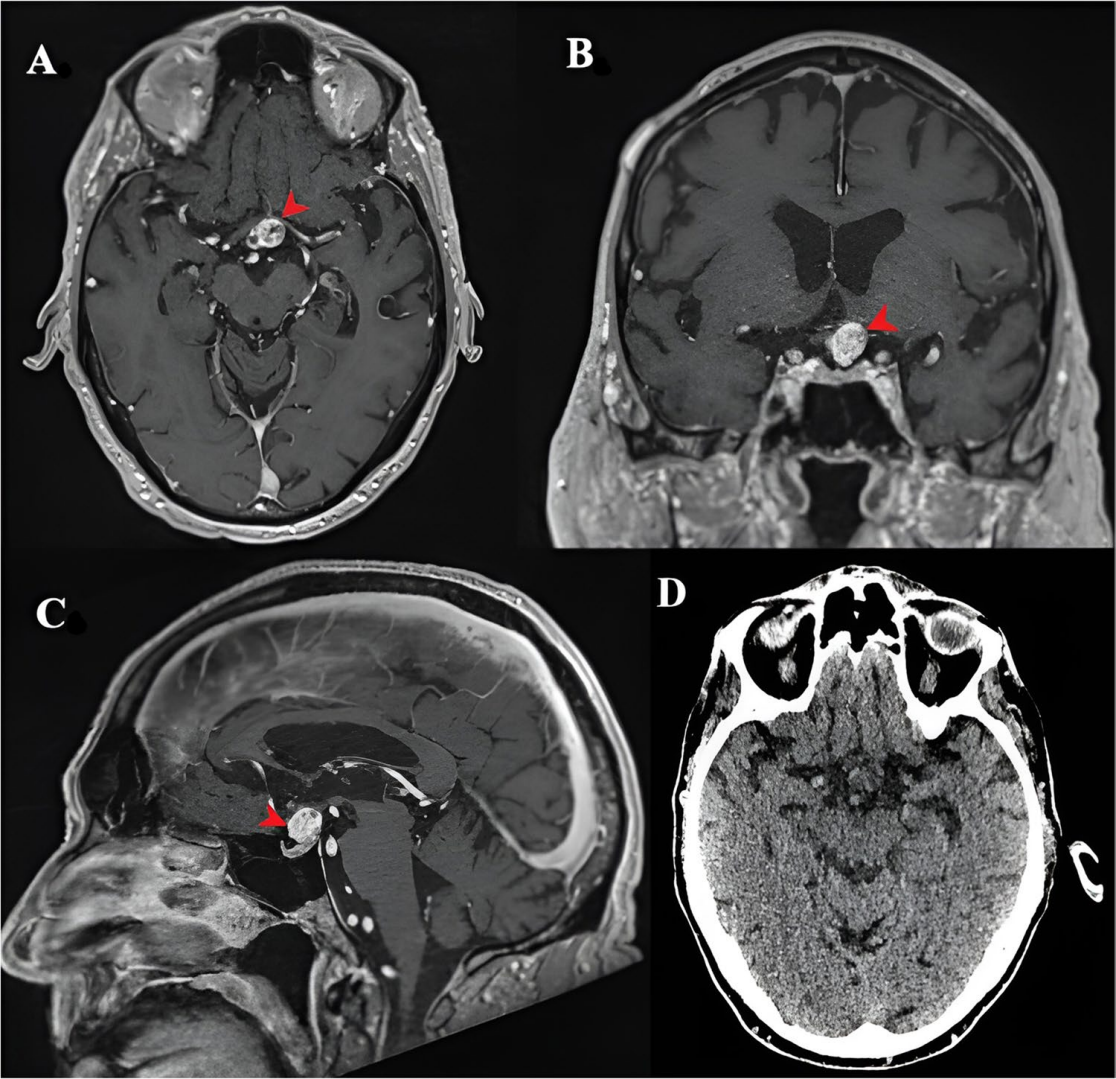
Preoperative imaging of Case 2: A to C.- Axial, coronal and sagittal T1 with contrast MRI images showing a solid enhancing lesion (red arrowhead) in the suprasellar space, D. CT image shows no calcification
